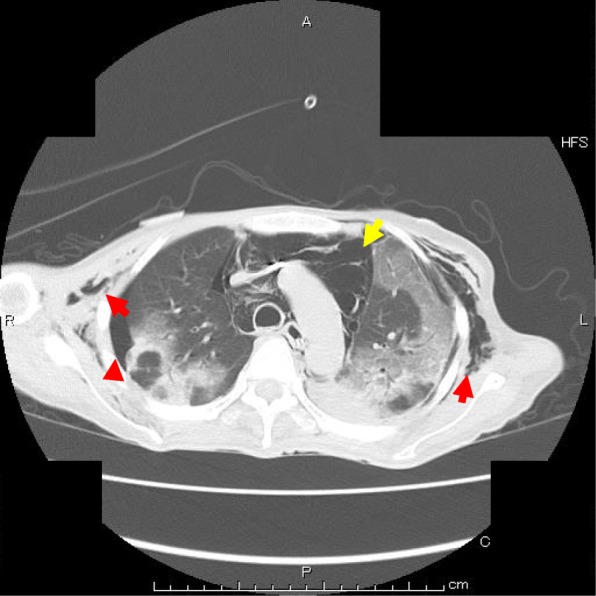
A subsequent chest CT following chest X-ray at deteriorated respiratory status. There are subcutaneous emphysema (red arrow), localized pneumothorax (red arrowhead), and pneumomediastinum (yellow arrow)
